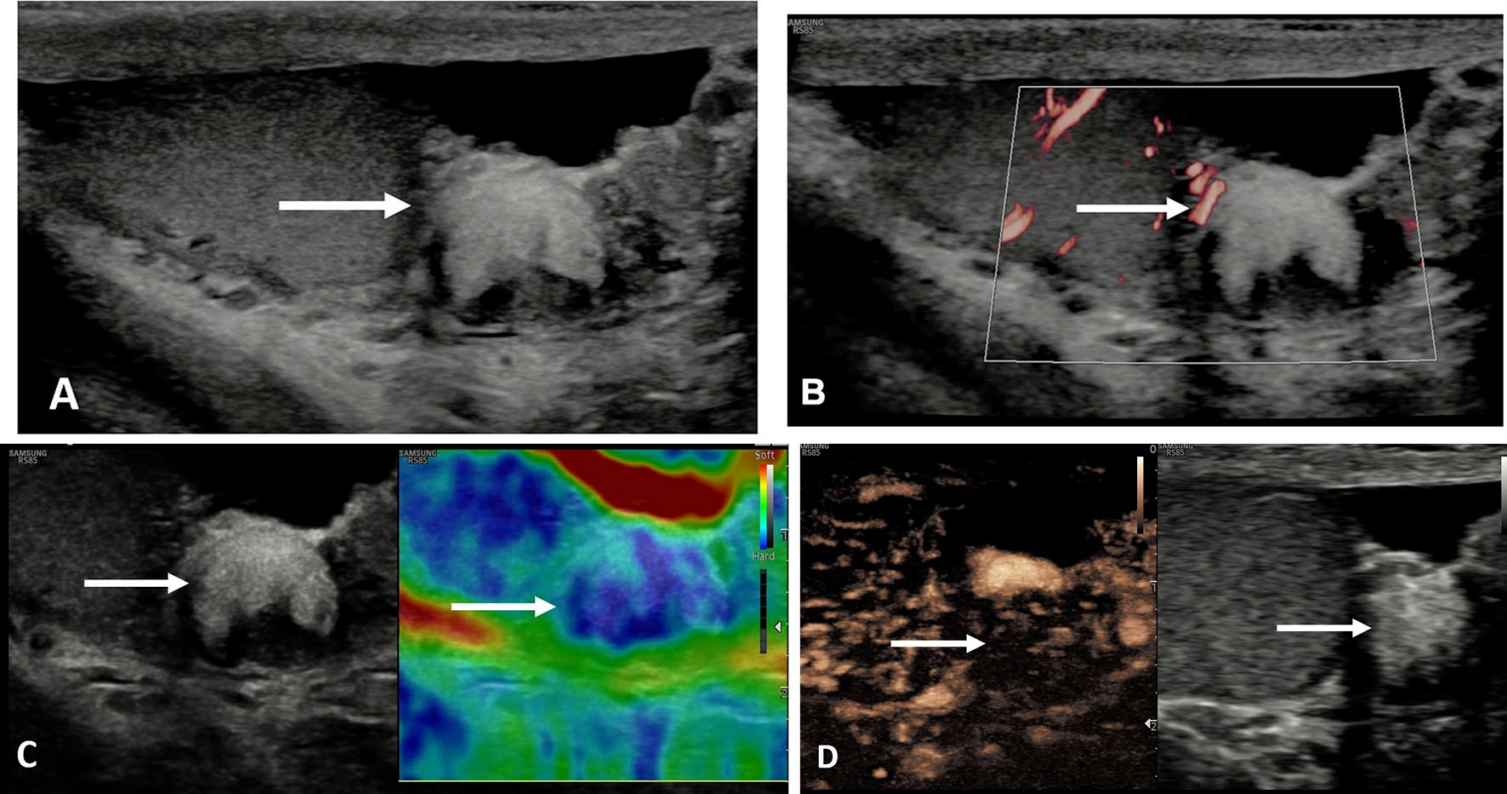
Palpable mass in the left epididymis in a 48-year old, with characteristics of a benign lipoma. A A longitudinal B-mode image of the left testis demonstrating an echogenic lesion in the epididymis (arrow). B Microvascular imaging demonstrates some vascular flow in the periphery of the lesion, but no central flow (arrow). C On elastography the echogenic lesion demonstrates increased stiffness (arrows). D On the contrast enhanced ultrasound examination, internal microbubble signal is observed (arrow) indicating some vascularization of the lipoma
